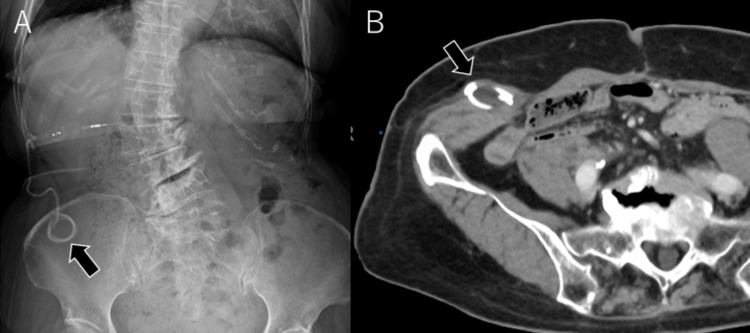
(A) An abdominal X-ray reveals coiling of the peritoneal catheter (arrow). (B) Abdominal CT shows migration of the peritoneal catheter into the subcutaneous tissue (arrow)
